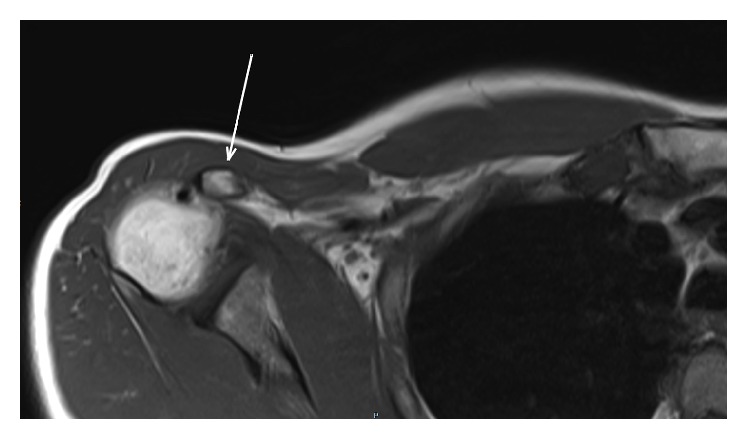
MRI axial T1 showing calcification inside the conjoint tendon immediately under its coracoid insertion.
